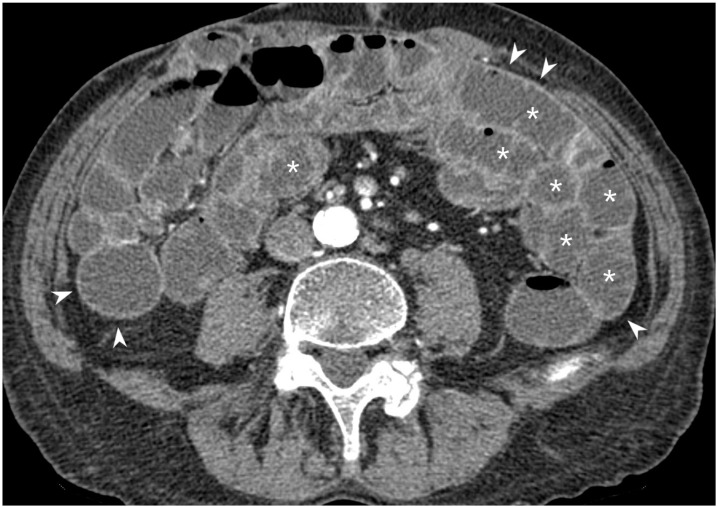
Axial CT-E image after iodinated contrast medium injection: example of intestinal loops’ distension by a hypodense contrast medium (polyethylene glycol solution), showing the hypodensity of the jejunal lumen (asterisks) and the hyperdensity of the intestinal wall (arrowheads). Original figures by LMM and LL.
